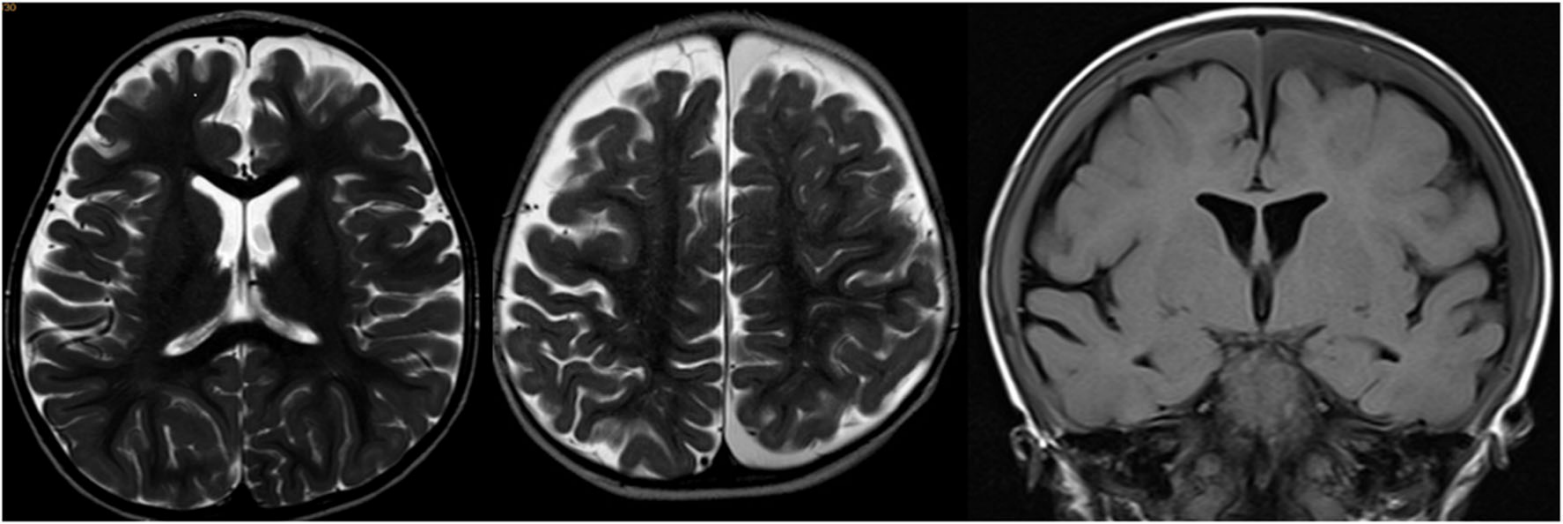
MRI images showing extra-cerebral fluid in the subarachnoid space (pat. #21—left), chronic haematomas localised in the left frontal and occipital subdural compartment (pat. #28—middle) and a globally distributed bilateral chronic SDH (pat. #13—right). Please note that the haematomas do not seem to compress the underlying cortex or the ventricles and that there is a brim of CSF between the haematomas and the cortical surface
